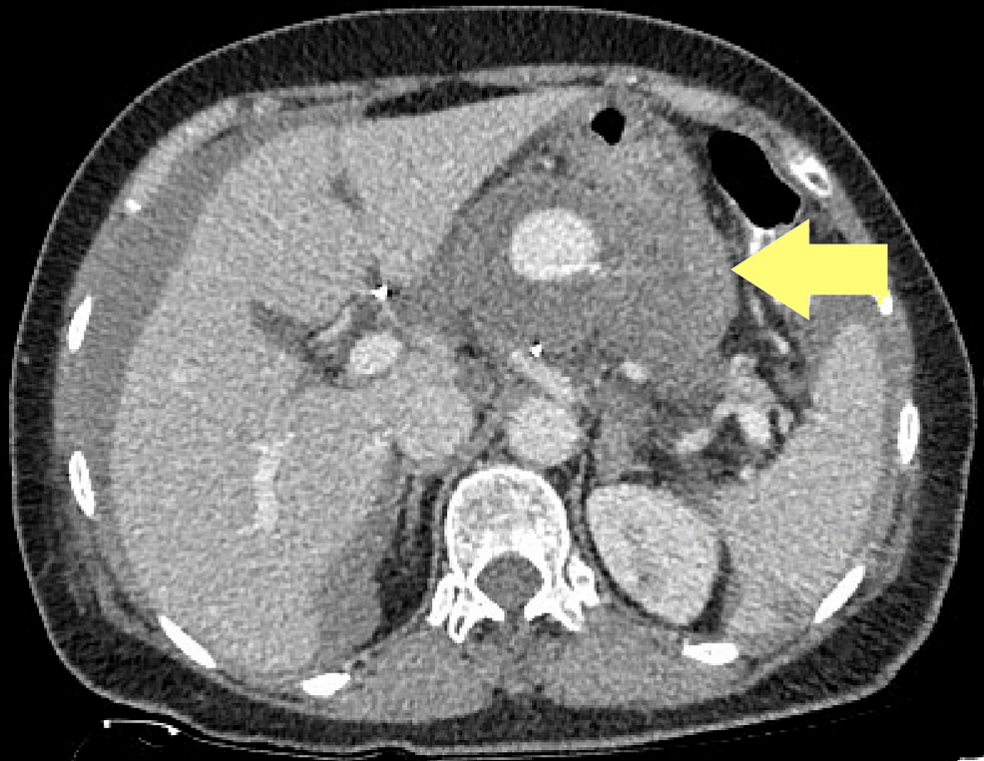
Preoperative CT angiography showing a partial rupture splenic artery aneurysm.
